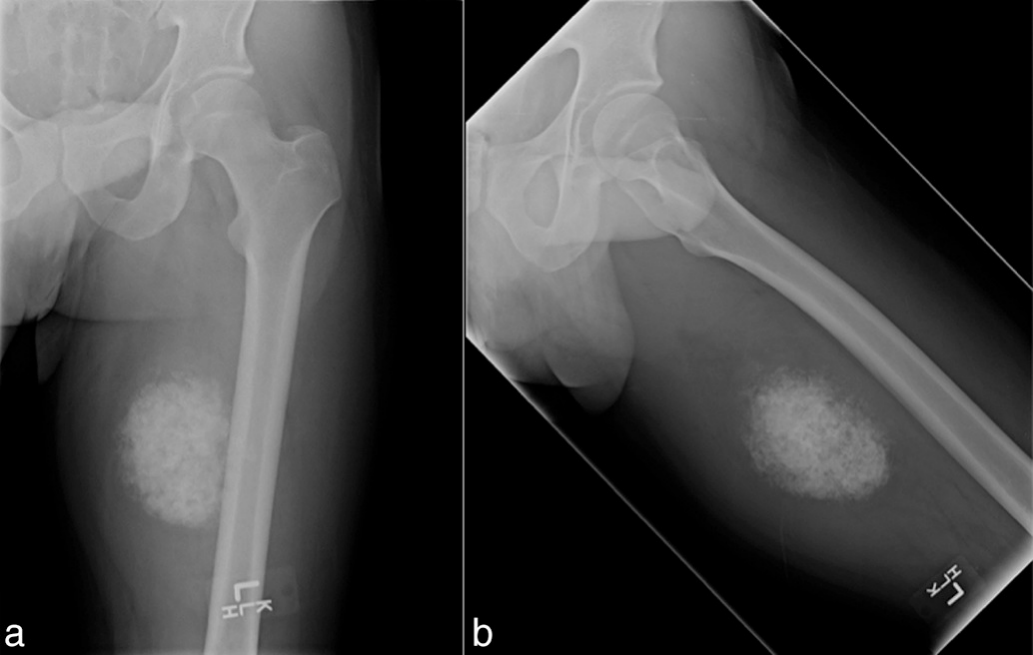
(a,b) Anteroposterior and lateral (frog leg) radiographs of the left femur demonstrate an extraskeletal lesion with dense minerlization, adjacent to the medial aspect of the femur.
